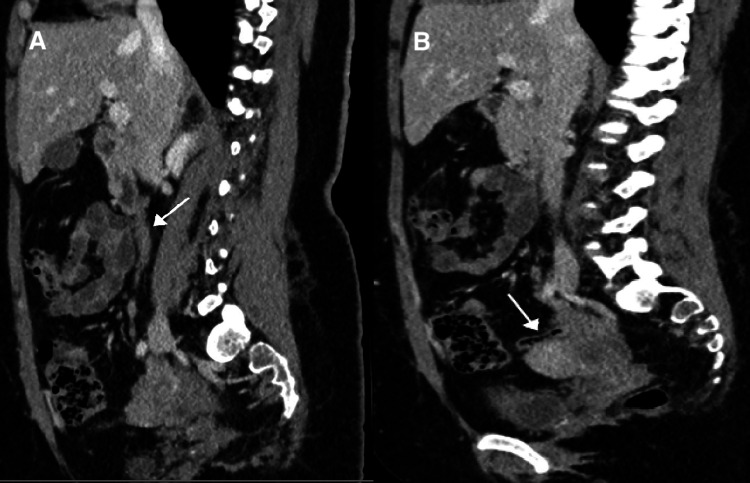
(A) Sagittal CT of the abdomen (venous phase) showing thickened tortuous right ovarian vein (white arrow). (B) Sagittal CT of the abdomen (venous phase) showing normal-looking appendix (white arrow). CT: computed tomography
